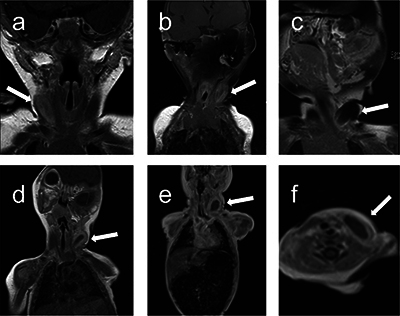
Contrast enhanced T1-weighted imaging, demonstrating the thick enhancing rim of the fibromatosis colli around a centre that remains isointense to the adjacent muscle (arrows). The coronal images (a) to (e) represent patients 1 to 5, with (f) being an axial reformatting of patient 5.
